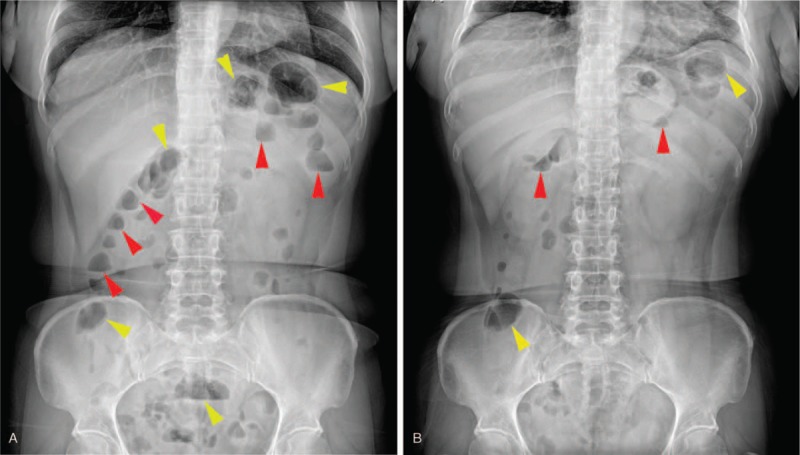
Abdominal plain radiography revealed amounts of air-fluid levels (red arrowheads) and massive gas accumulation in the dilated intestinal canal (yellow arrowheads) before glucocorticoid treatment (A), and fewer air-fluid levels (red arrowheads) and massive gas accumulation in the dilated intestinal canal (yellow arrowheads) after glucocorticoid treatment (B).
